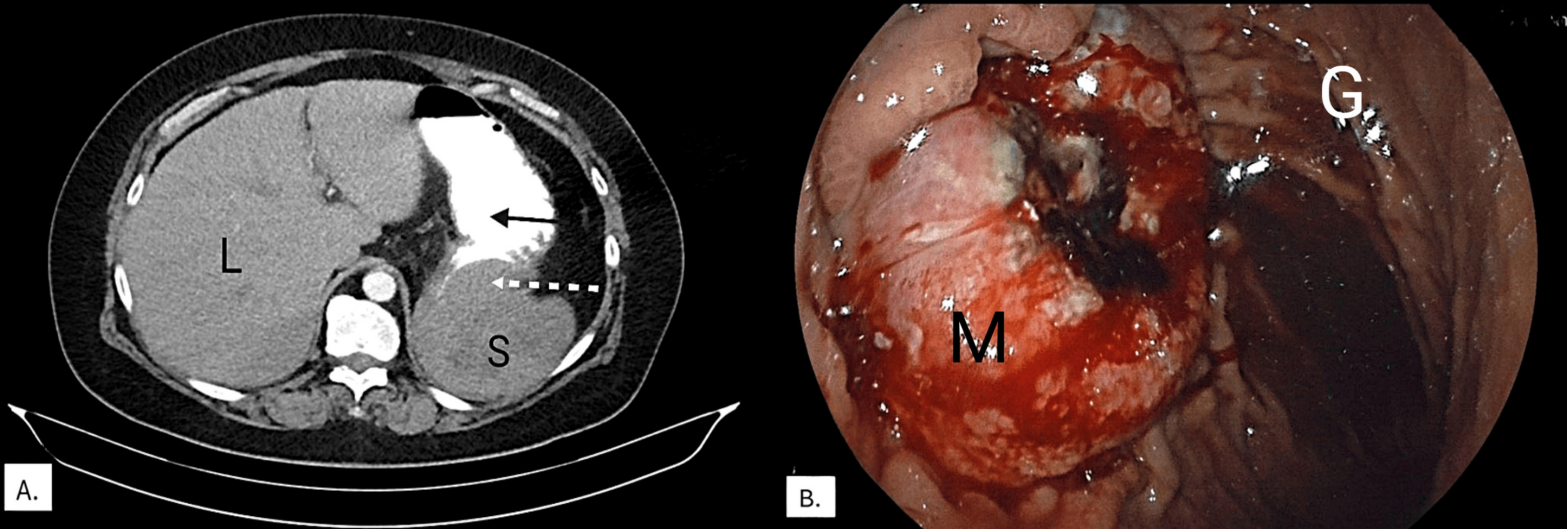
Imaging and endoscopic findings. A. Abdominal CT with IV and PO contrast shows a large splenic mass measuring 6.8 x 6.8 cm invading the gastric fundus. L: liver; S: spleen; Black arrow: stomach after oral contrast ingestion; White dashed arrow: splenic mass infiltrating the gastric fundus. B. Gastroscopy shows a slowly oozing, ulcerated lesion measuring 6 x 6 cm, representing full-thickness wall infiltration. M: splenic metastasis fully invading the gastric wall; G: normal gastric mucosa.
